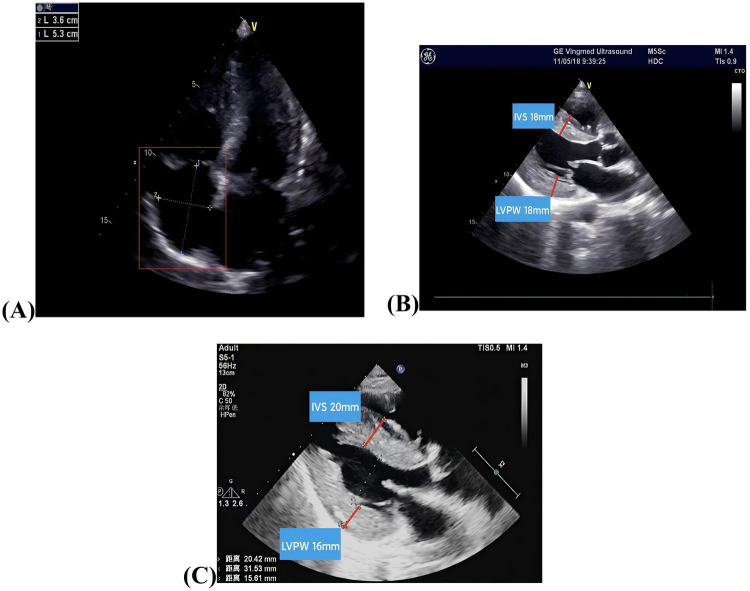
Dynamic echocardiographic changes in the patient with Fabry disease. (A) Echocardiography in 2018 shows symmetric left ventricular hypertrophy. (B) Echocardiography in 2018 shows interventricular septum and left ventricular posterior wall thicknesses, with both measuring 18 mm. (C) Echocardiography in 2025 shows septal thickness progression (basal septal thickness 20 mm).
